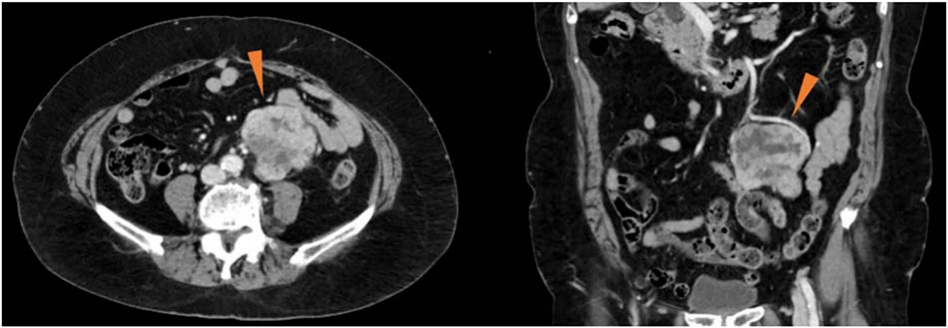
Contrast-enhanced computed tomography showing a well-defined, heterogeneously enhanced mass (red triangles) in the vicinity of the left ovarian artery and left ureter.
